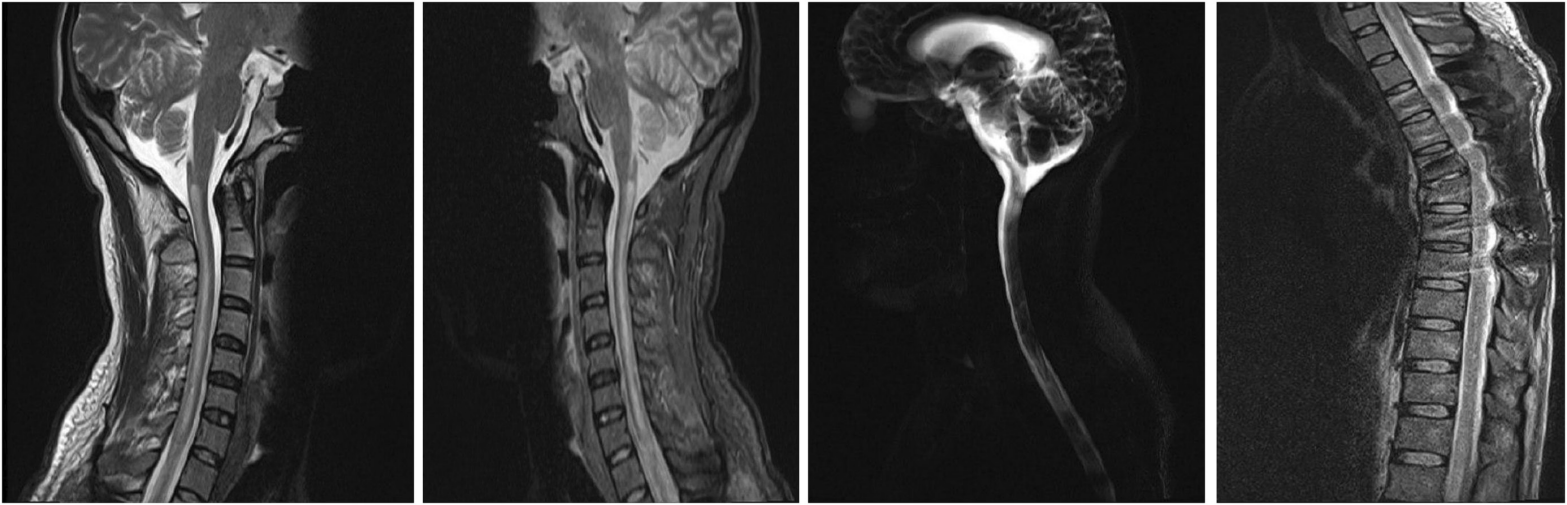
Follow‐up MRI of the patient in cervical and thoracic sequences after 50 days
